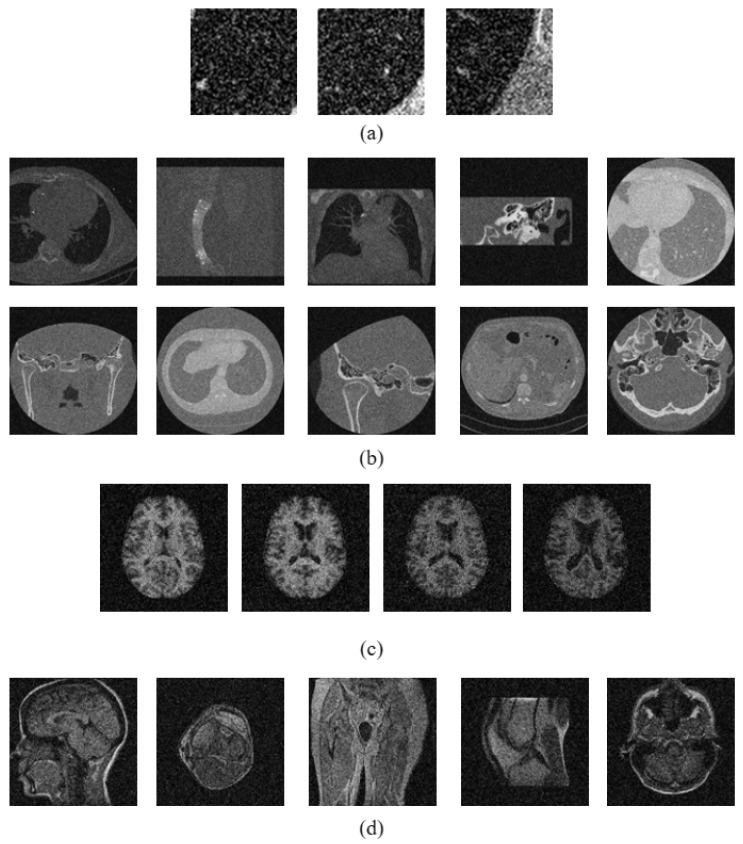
Sample noisy image from each class of (a) Emphysema CT database, (b) NEMA CT database, (c) OASIS MRI database, and (d) NEMA MRI database.
